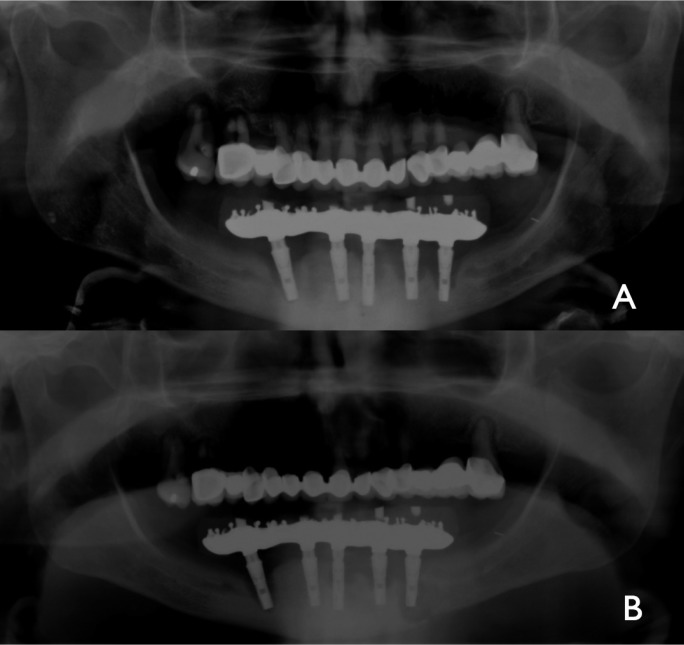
Case 2: A) showing PI around most of the implants. B) Panoramic radiography revealing pathological mandibular fracture around left distal implant.
